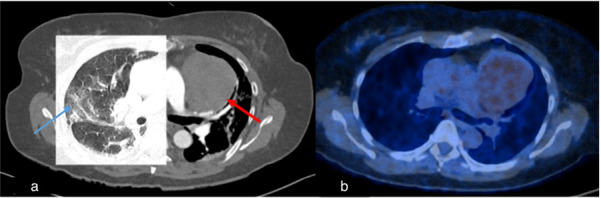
Case 2, 60-year-old female. (a) Initial chest CT to clarify pulmonary embolism or COVID-19 findings showed pneumonic infiltrates of indeterminate appearance for COVID-19 (blue arrow) and revealed a large mediastinal mass (red arrow). (b) PET/CT demonstrated mild FDG-uptake, making the diagnosis of thymoma more likely than lymphoma.
